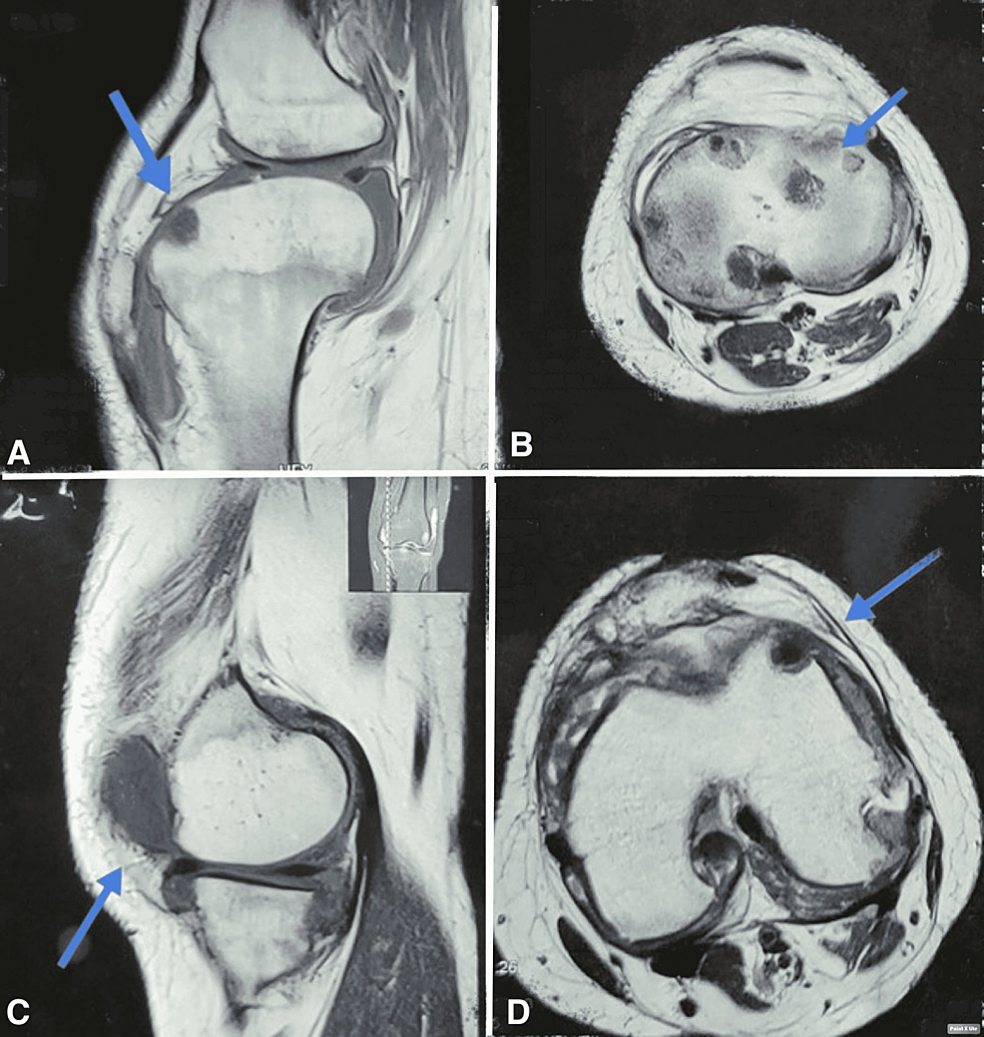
T1/T2-weighted MRI images of knee joint. Coronal sections (A,C) and axial sections (B,D) show well-defined lucency in patella and surrounding area involving cartilage and bony tissue.
